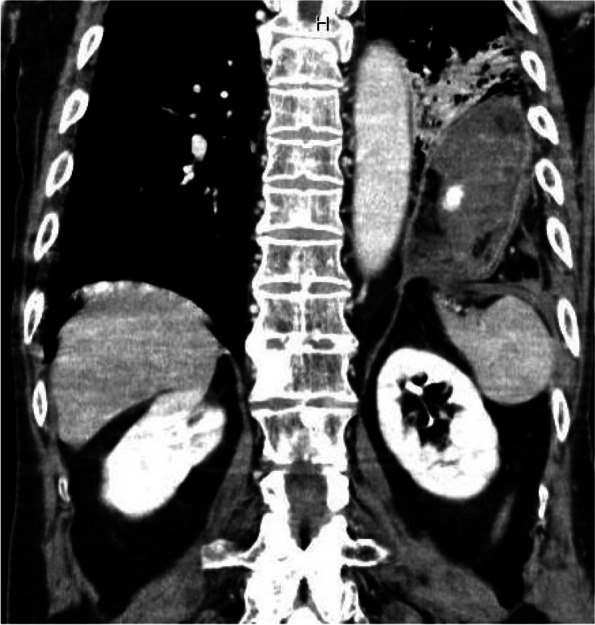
Preoperative chest computed tomography scans showing left diaphragmatic injury with hernia
